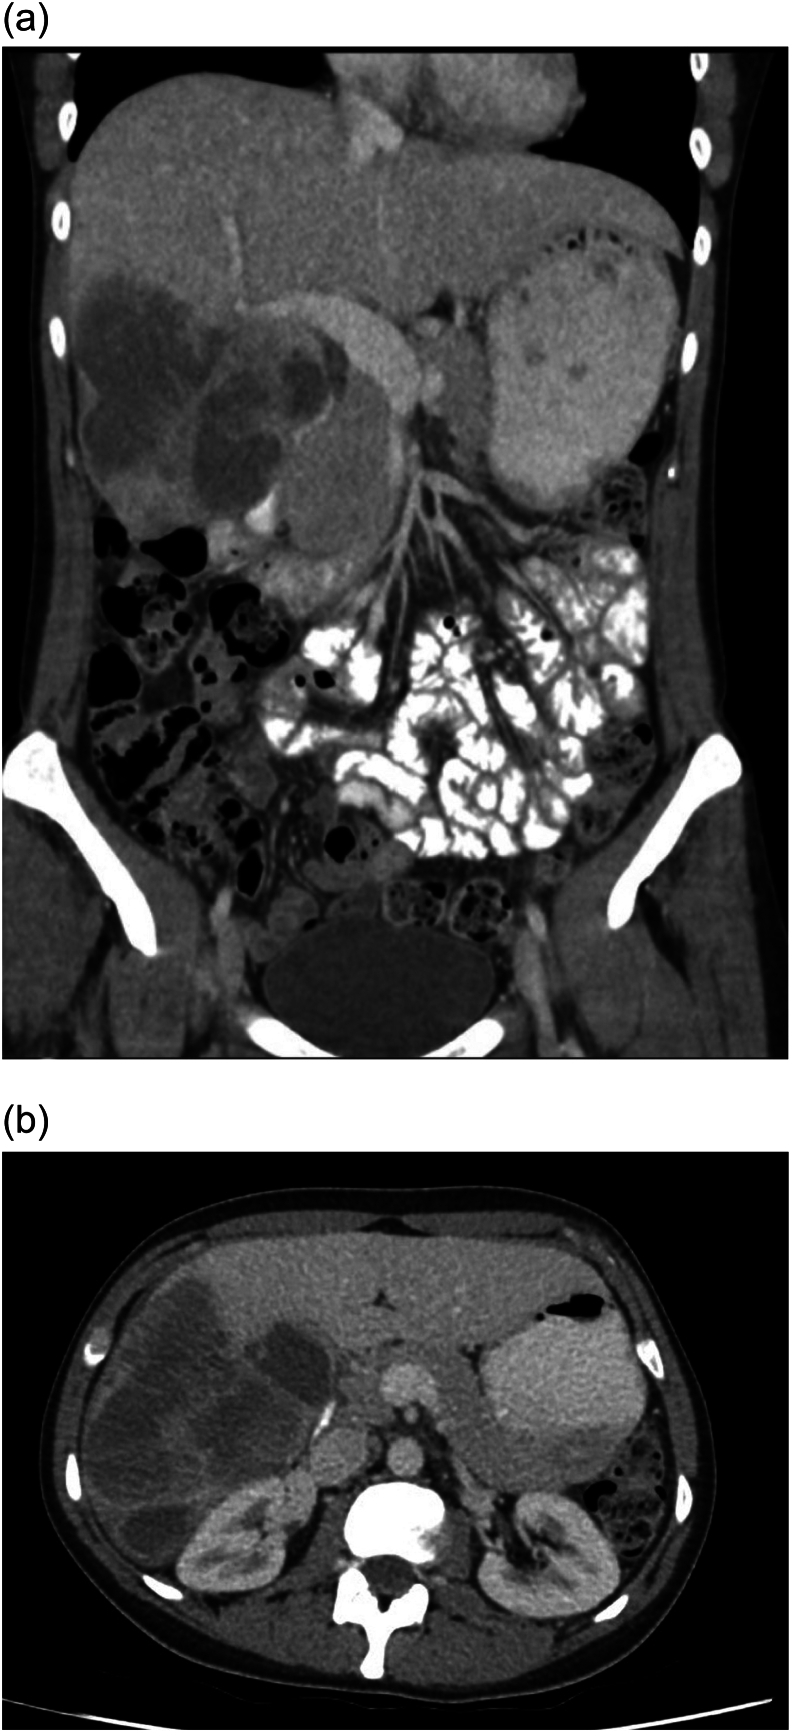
CT liver - coronal & axial view.
